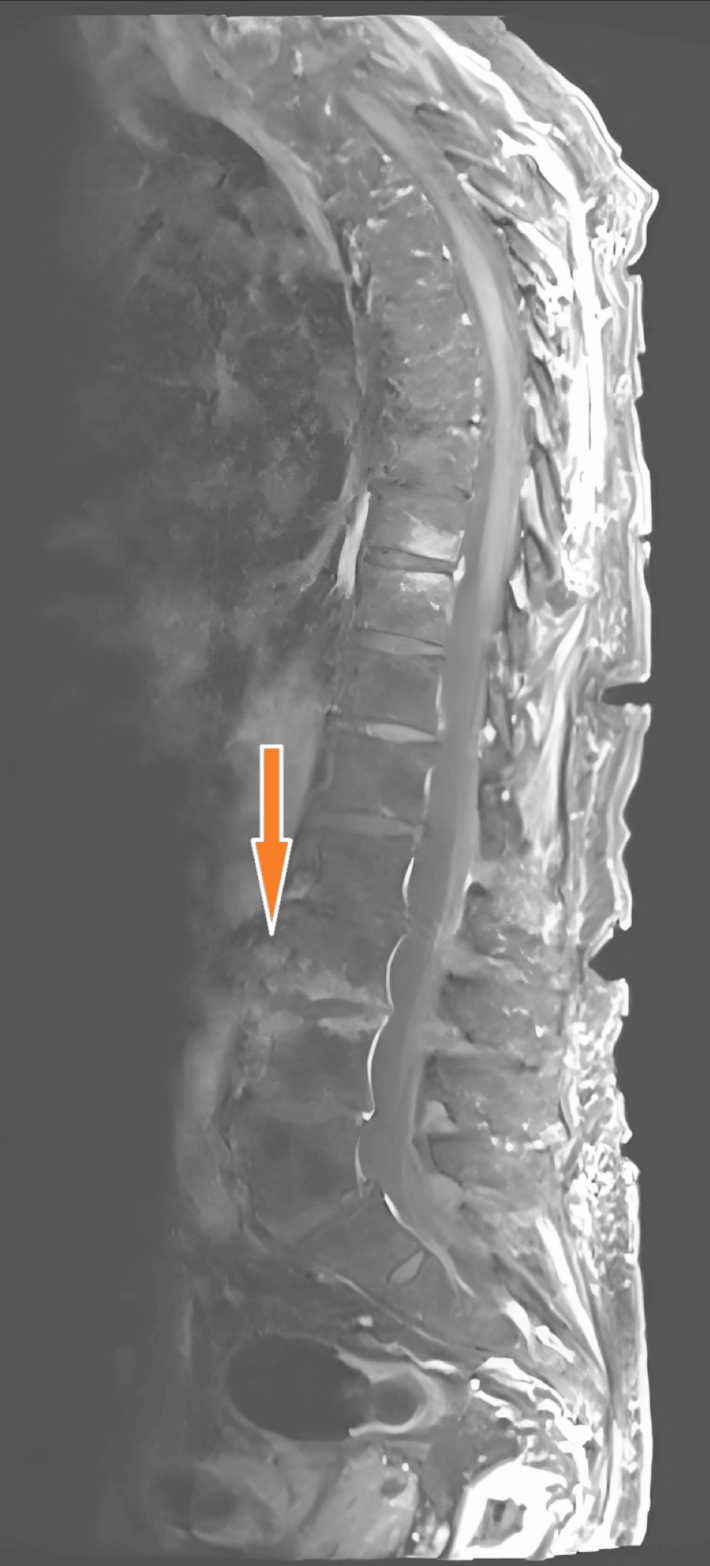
Sagittal MRI of the lumbar spine showing inflammatory changes and destruction at the L2/L3 intervertebral space, consistent with spondylodiscitis. No intraspinal abscess formation was observed. The orange arrow indicates the site of vertebral involvement.
